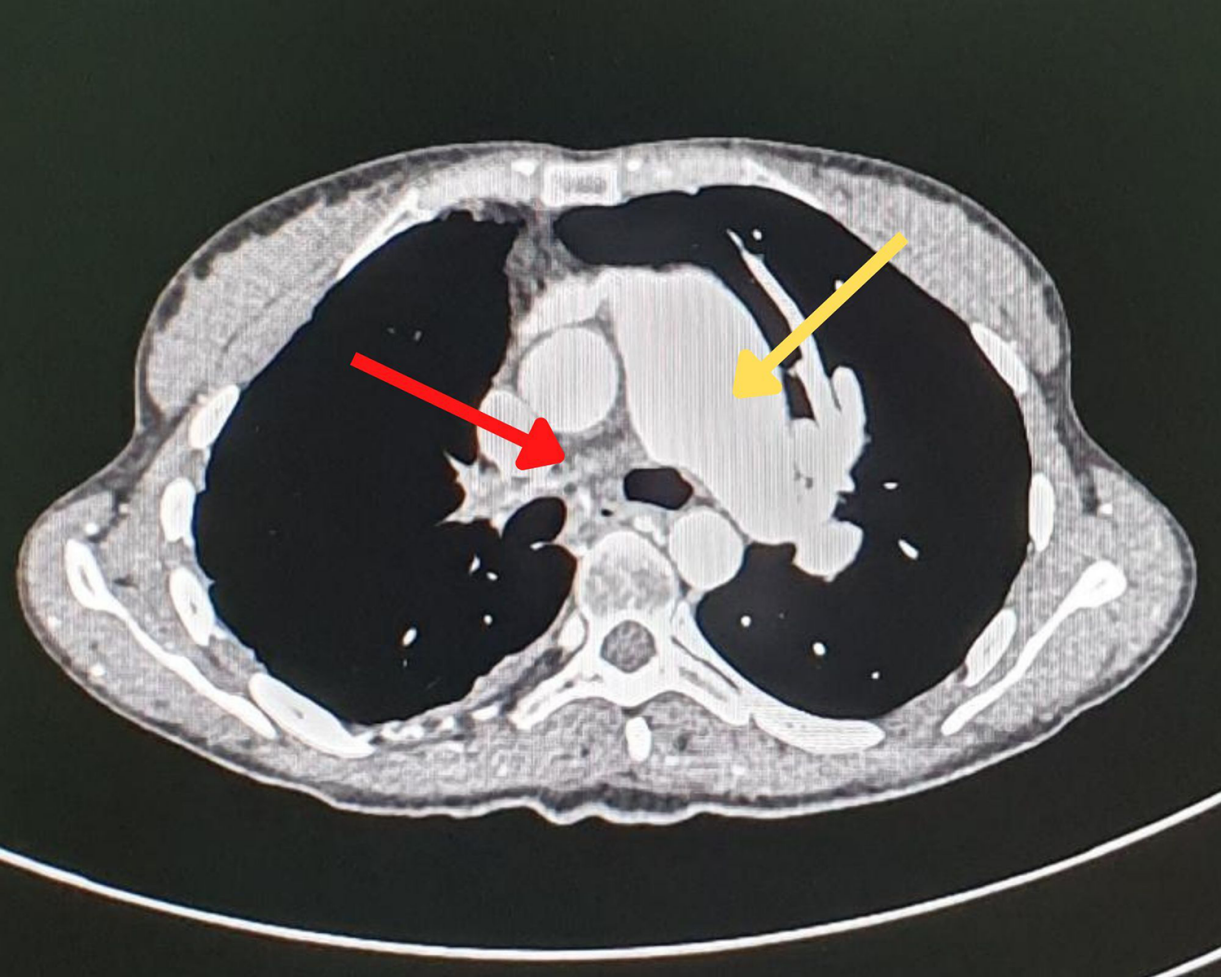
Chest angiographic CT showing a dilatation of the main pulmonary artery (yellow arrow) and a lack of the opacification of the right pulmonary artery (red arrow), consistent with a severe stenosis of the latter.
